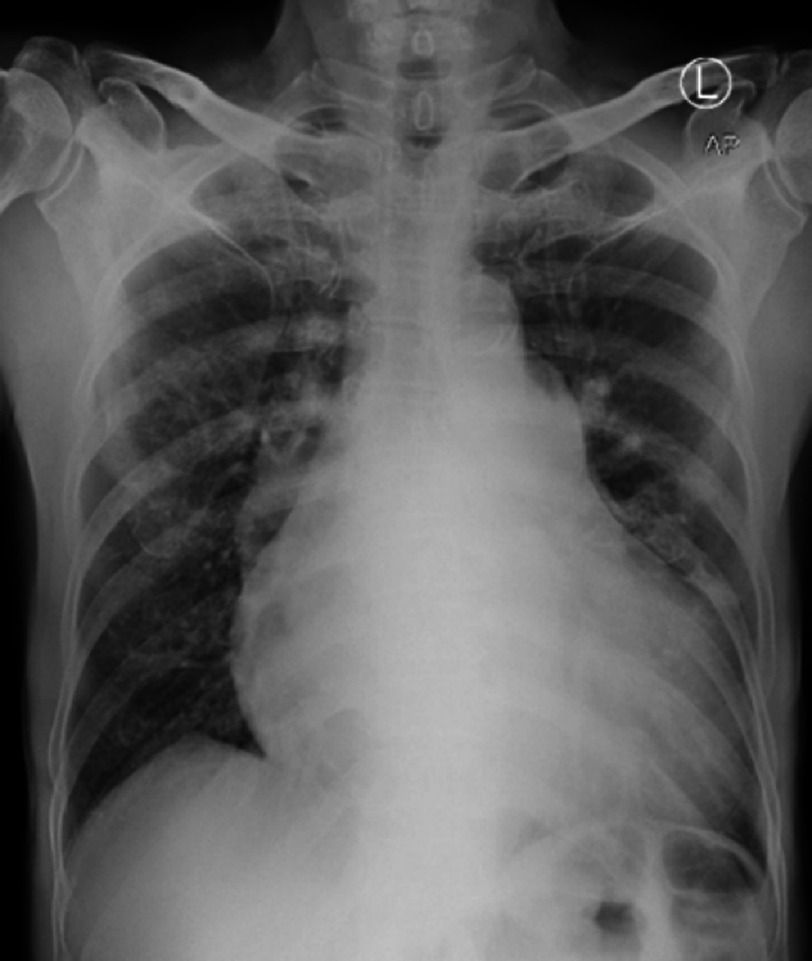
Postero-anterior chest X-ray.
